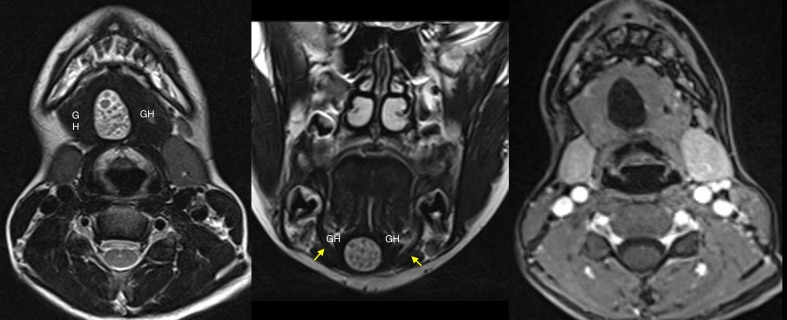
Magnetic resonance imaging (MRI) Axial T2-weighted-MRI (left panel) demonstrates a well-defined cystic mass between the geniohyoid muscles (GH); intracystic low-in-signal free bodies are also visualized. Coronal T2-weighted-MRI (middle panel) depicts the sublingual location of the cyst which lies above the mylohyoid muscle (arrows) between the GH. The cystic lesion does not enhance on post-contrast MRI (right panel); the intracystic corpuscles are faintly visible.
